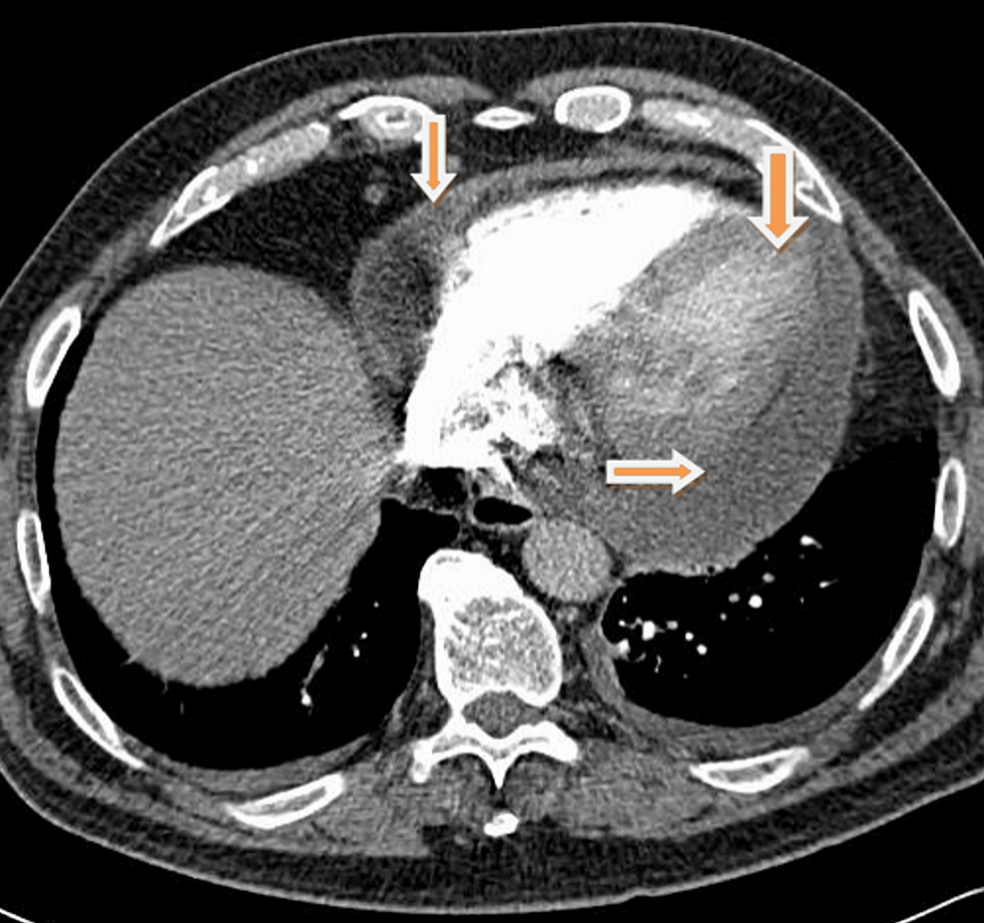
CTPA with pericardial effusion indicated CTPA: computed tomography angiography
